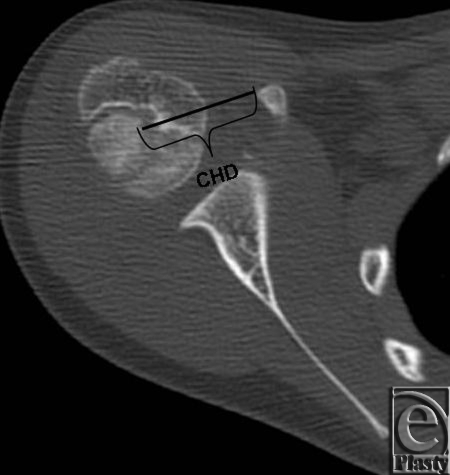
Representative axial computed tomographic image of the affected shoulder from a patient with obstetric brachial plexus injury. CHD represents the coracohumeral distance, measured from the coracoid process to the center of the humeral head.
